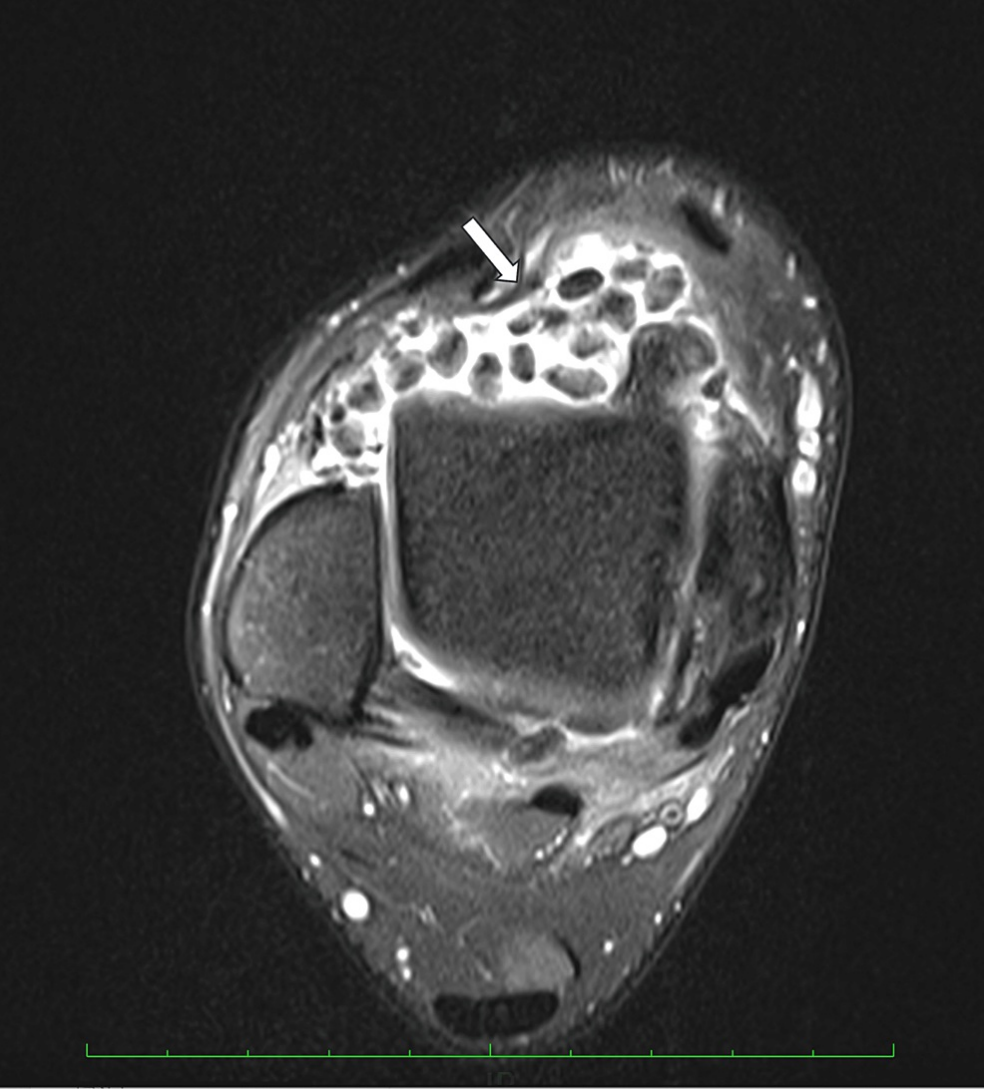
Preoperative axial dual phase MRI of the right ankle.
